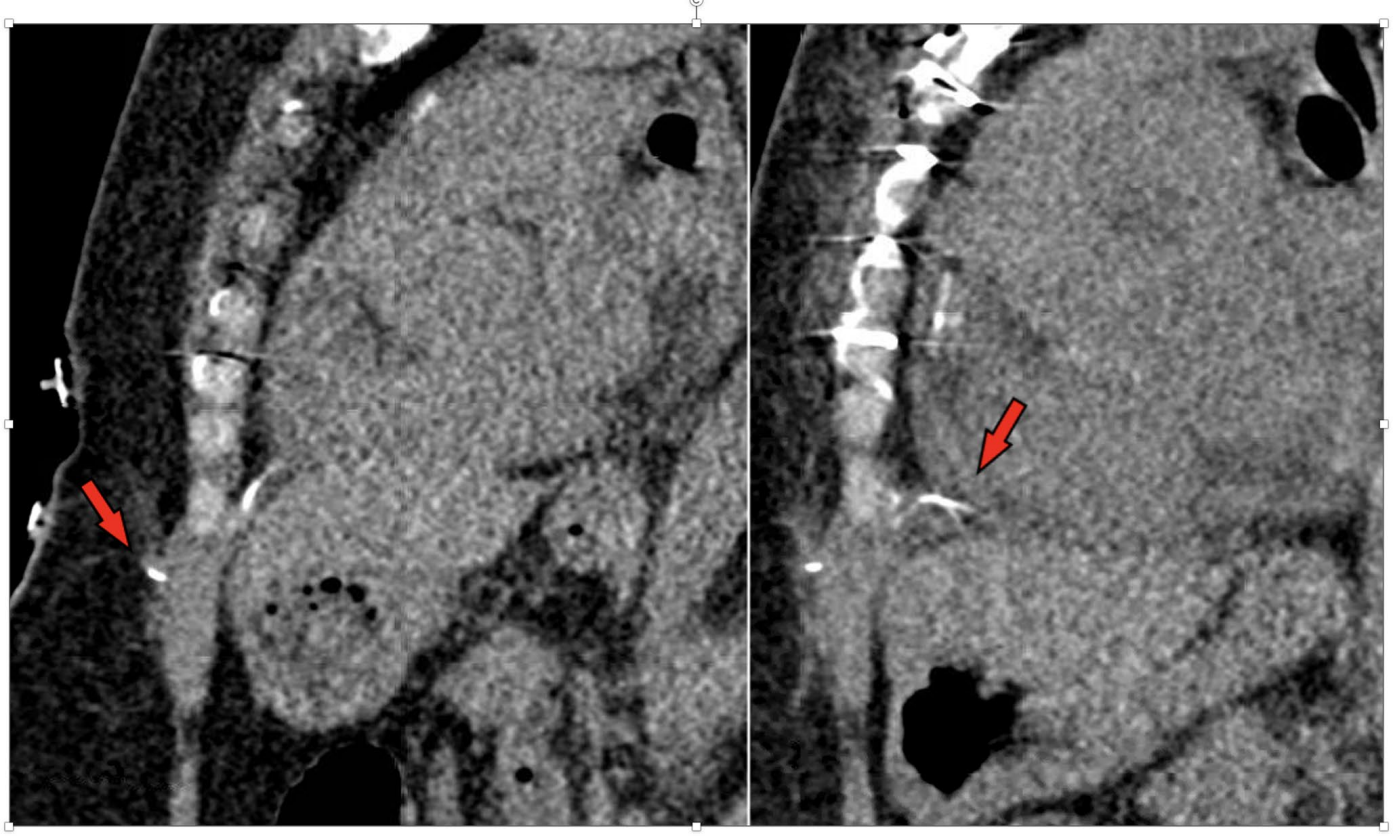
Non-contrast chest CT sagittal reconstructions for the patient case with minor skin sensation reaction during cervical spine MRI. Arrows demarcate the truncated epicardial pacer lead in the subxiphoid soft tissues and terminal abutting the anterior heart (red arrows). Partially visualized additional epicardial pacing wires over the right atrium. There are expected post-surgical changes about the anterior body wall. No fluid collection or reactive changes in the soft tissue are noted. There was no pericardial effusion. The leads were noted to be in an unchanged position in comparison with a prior chest CT
